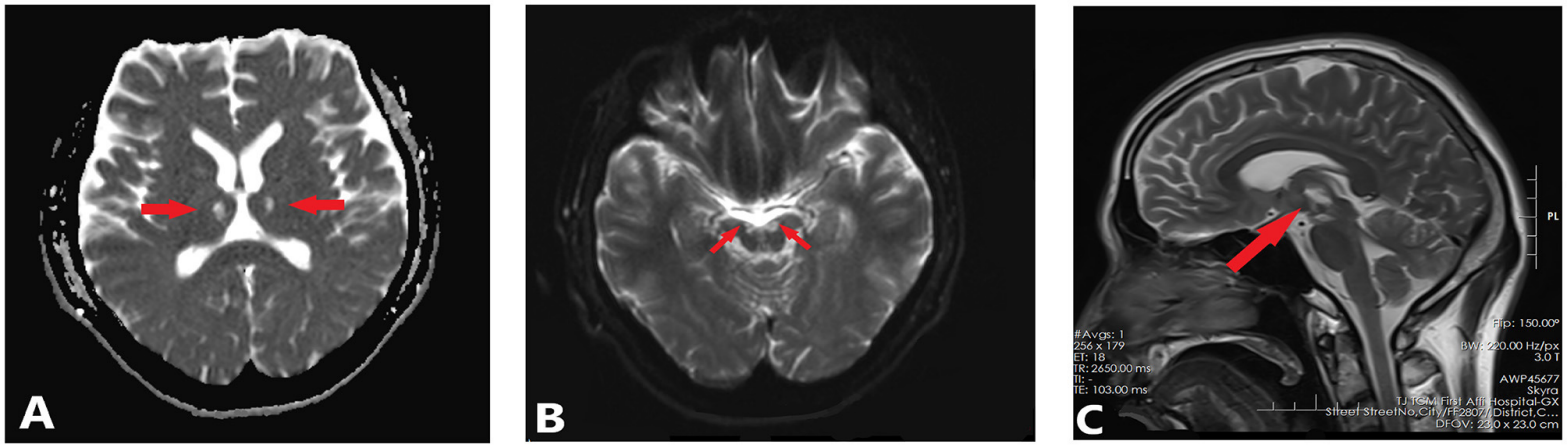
MRI revealed abnormal signals in the midbrain and thalamus. (A) The axial scanning of MRI revealed bilateral thalamic infarcts (DWI, diffusion-weighted imaging). (B) The axial scanning of MRI revealed Mesencephalic infarction (DWI, diffusion-weighted imaging). (C) The sagittal scanning of MRI revealed Mesencephalic infarction(T2-weighted MR images).
